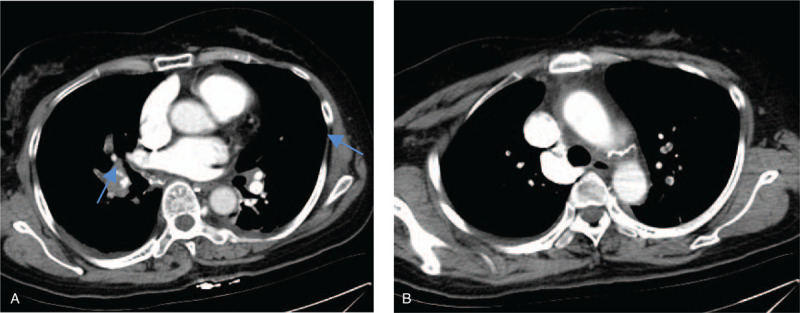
a,b: pulmonary CTA, showing multiple pulmonary embolism in the trunk of the right lower pulmonary artery and the branch of the left lower pulmonary arteries.
